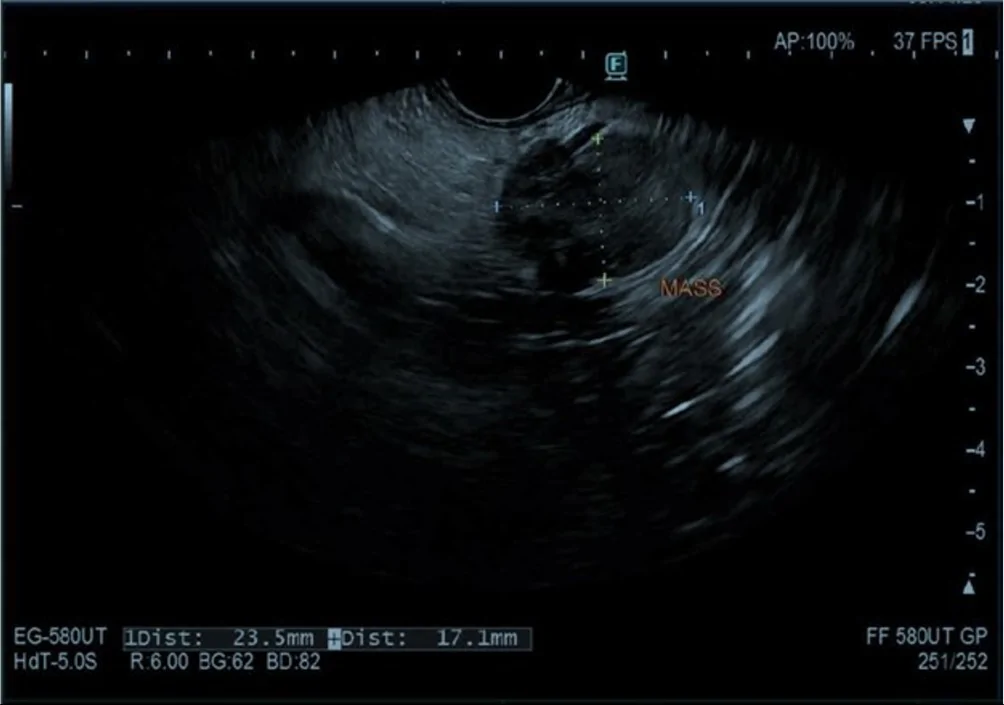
Endoscopic ultrasound of index pancreatic lesion. Hypoechoic mass of the pancreatic head measuring 23.5 mm × 17.1 mm.
